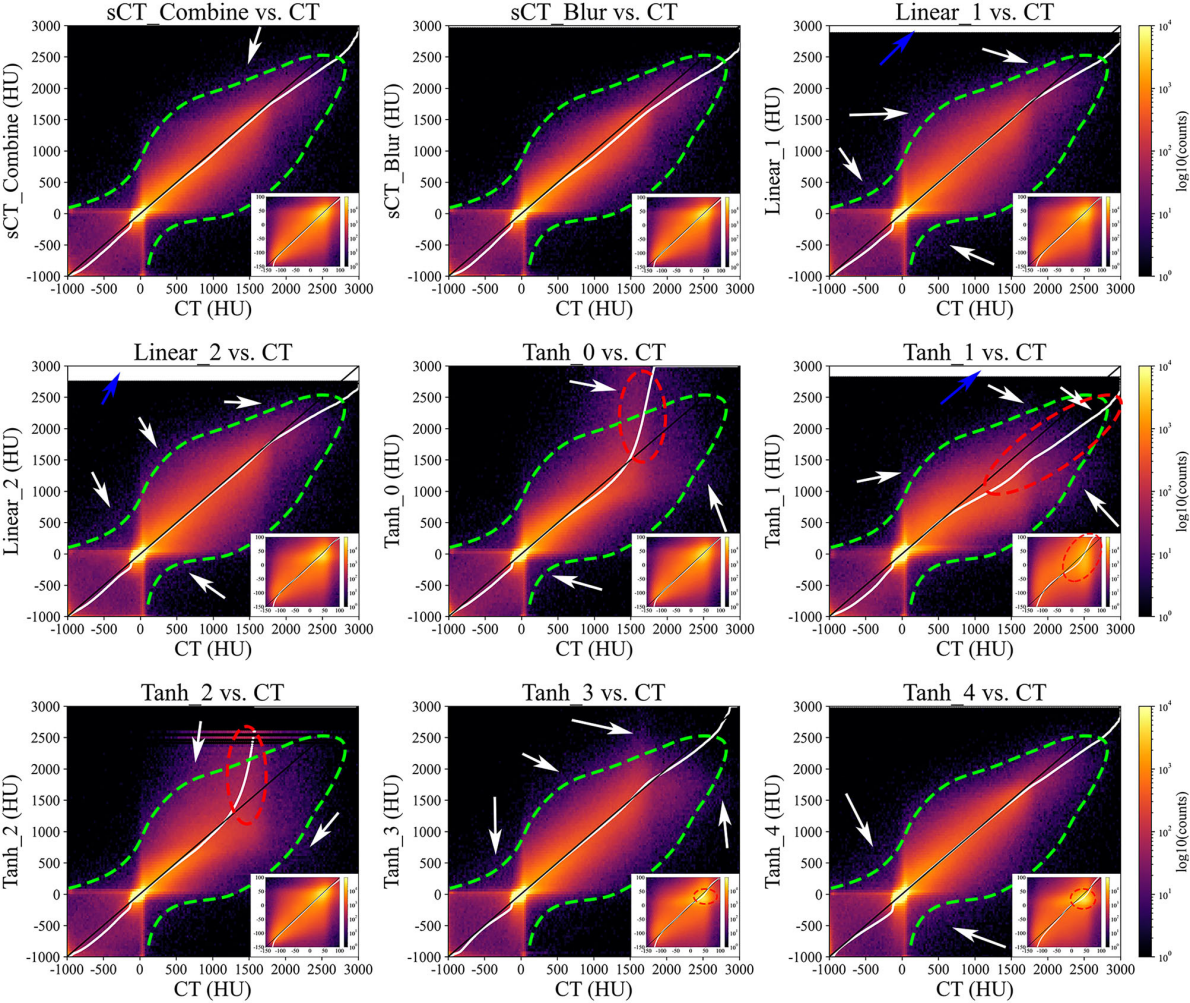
Two‐dimensional histograms and Q‐Q plots of the sCT_Combine, sCT_Blur and uncombined sCT images relative to the planning CT images in the range of [−1000, 3000] HU. The brighter the color near the 45‐degree line and the more concentrated the bright color block, the more accurate the CT number of the sCT image and the smaller the dispersion of the CT number error. The white curve is the Q‐Q plot. If the Q‐Q plot deviates too much from the 45‐degree line, it indicates that the CT number distribution of the two images has a large difference. To better compare the soft tissue regions of the images, 2D histograms were redrawn and magnified in the lower right corner of each subplot in the range of −150 to 100 HU. The green dashed lines in the subfigures indicates the main concentration area of brighter blocks in sCT‐Blur. CT, computed tomography; sCT, synthetic computed tomography.
